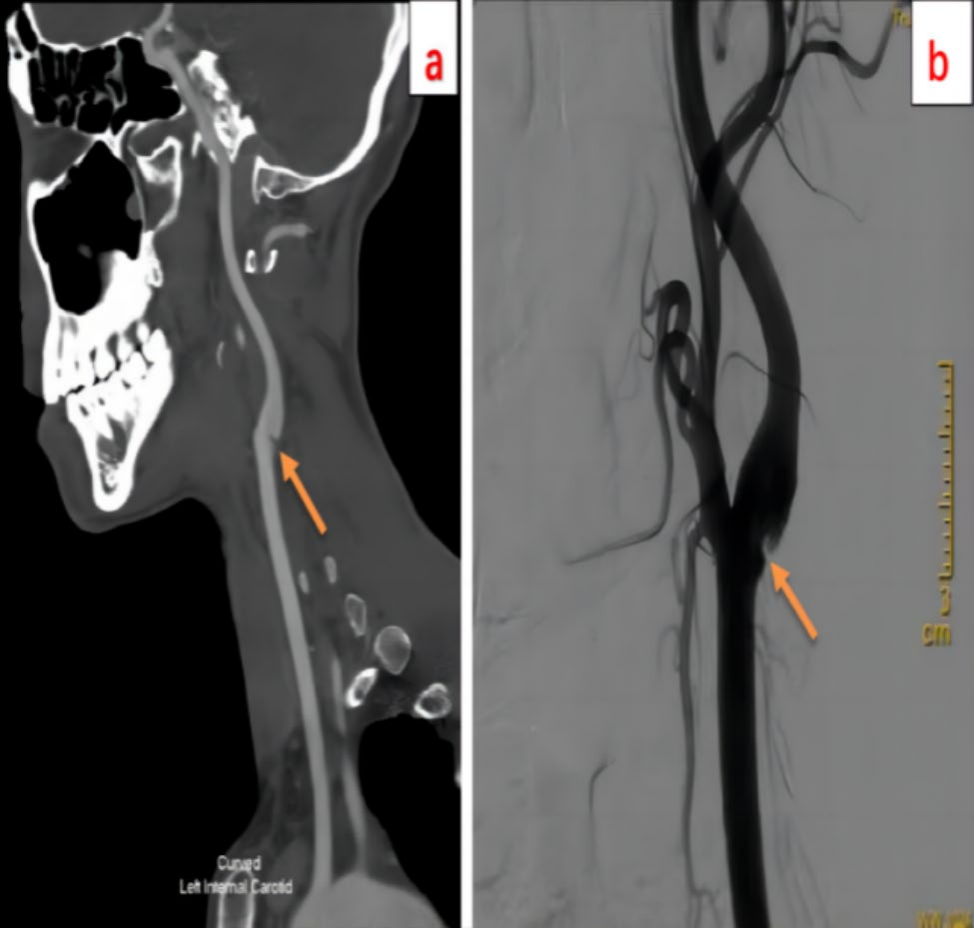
a,b CTA and DSA showed CaW (orange arrow), no filling defect
